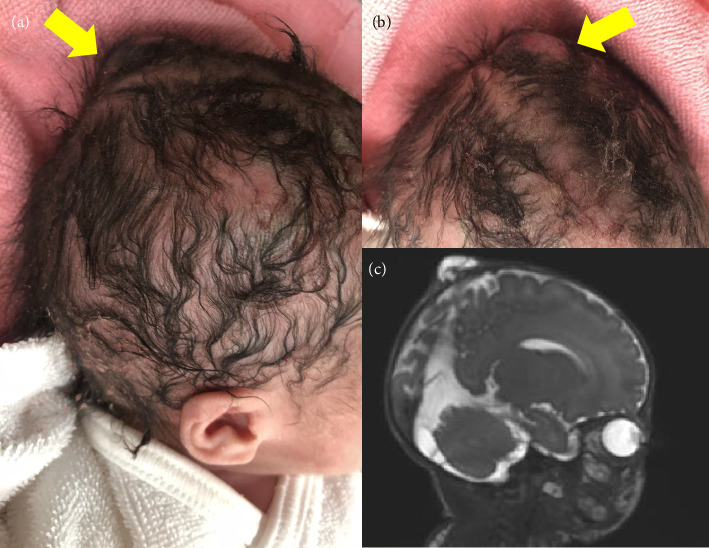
Gross findings and postnatal magnetic resonance imaging (MRI) of the scalp cyst. (a) Lateral view of the scalp cyst (yellow arrow). (b) Frontal view of the scalp cyst. A small lesion is observed (yellow arrow). (c) Lateral view of the small scalp cyst connected to the intracranial space observed using T2-weighed MRI.
